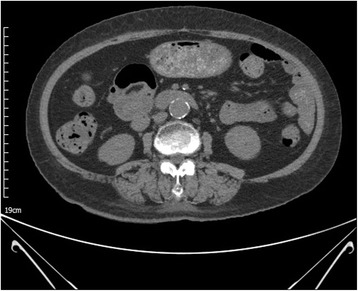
Follow-up abdominal CT after 2 week shows nearly resolved state of PCI
